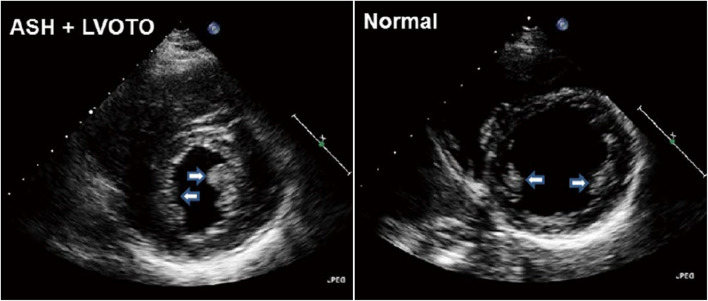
Representative parasternal short axis views of two-dimensional echocardiography demonstrating different papillary muscle positions in hypertrophic cardiomyopathy. Compared with controls, papillary muscles in hypertrophic cardiomyopathy frequently showed a more anteriorly displaced position
